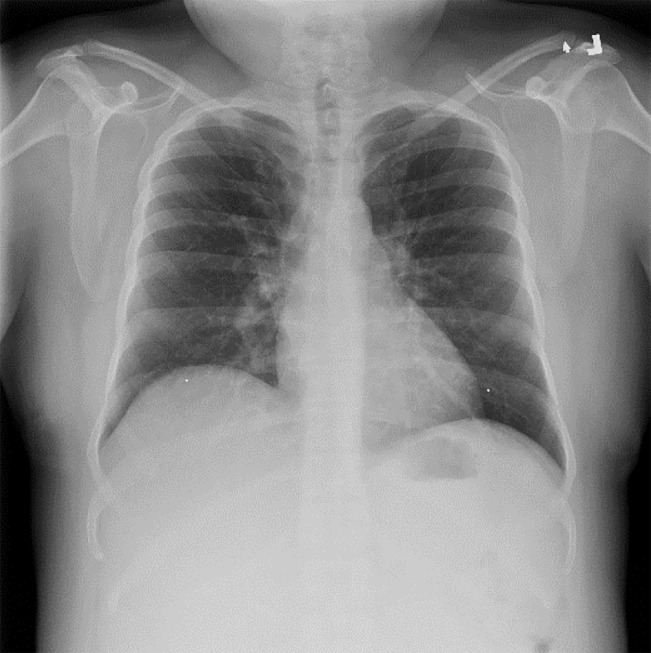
Anterior-posterior chest radiograph of a 37-year-old transgender male with sudden decompensation while in the emergency department.
